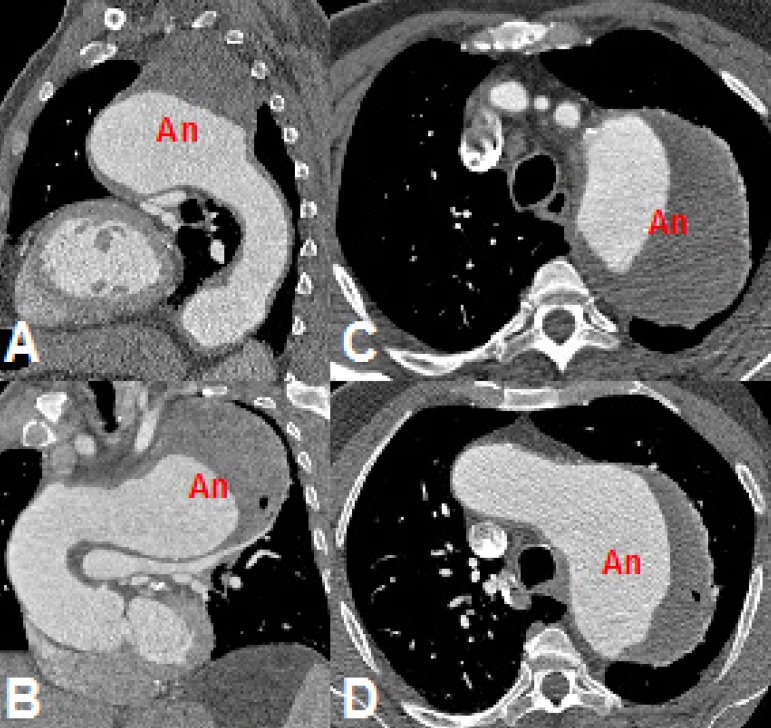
Computed tomography (CT) angiography of the 10 cm thoracic aortic
